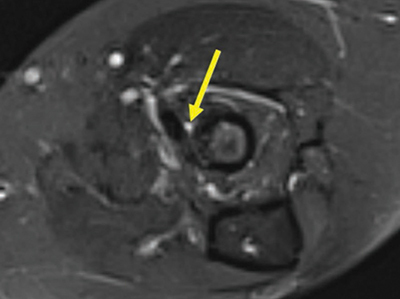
Axial fat-saturated T2-weighted image at the level of the biceps tendon insertion with a T2-hyperintense signal next to it, score 1.
